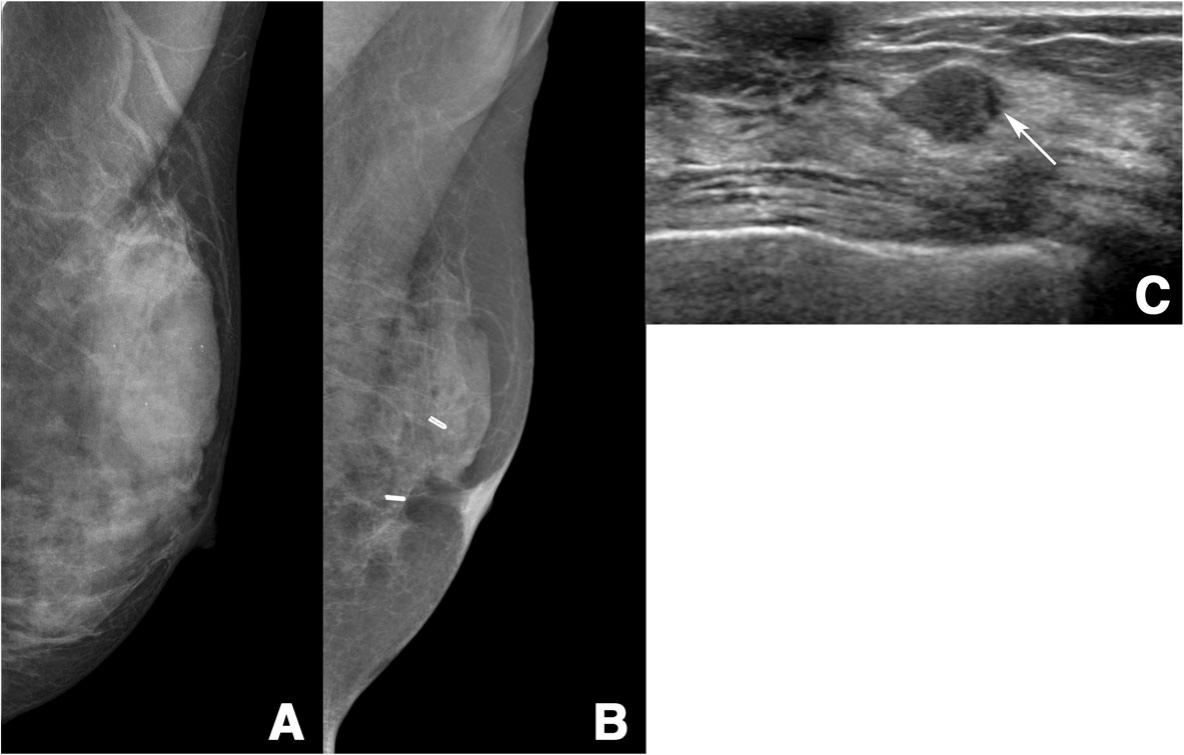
A 48-year-old woman with a PHBC in the left breast, classified as ‘true negative’. a Mediolateral oblique mammogram at primary breast cancer diagnosis showed extremely dense breast tissue and benign calcifications that were interpreted as negative. Primary breast cancer was a 22-mm microinvasive ductal carcinoma in the left breast. b Mediolateral oblique mammogram obtained 43 months after surgery also shows extremely dense breast tissue and no detectable abnormality except a postoperative change in the left breast. c Ultrasound image shows an 11-mm hypoechoic mass with partially not circumscribed margin (arrow) in the left breast subareolar area, which was pathologically proven to be a 12-mm microinvasive ductal carcinoma
